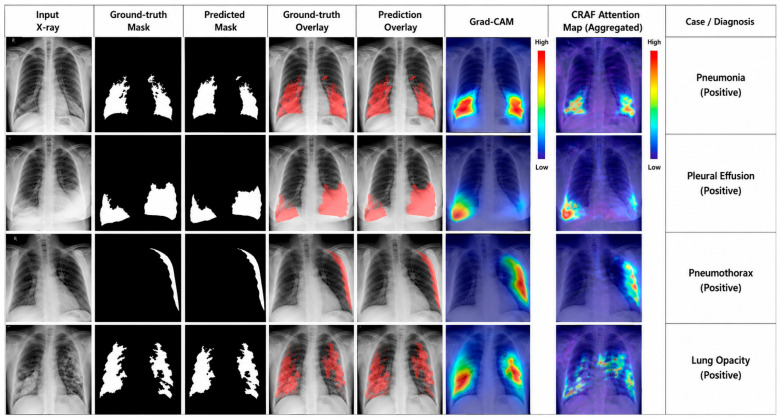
Qualitative chest X-ray results.
